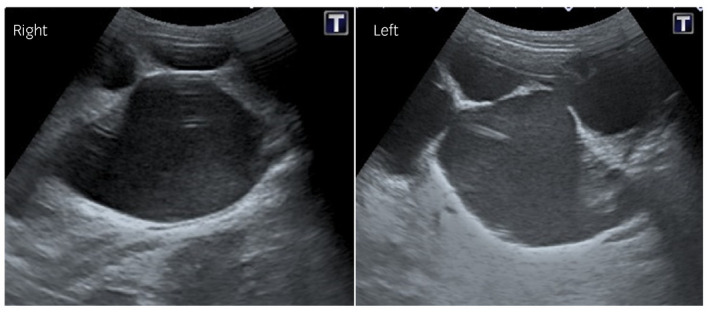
Intraoperative ultrasonography showing severe right and left dilatation of the renal pelvis and calyx with thinning parenchyma.
